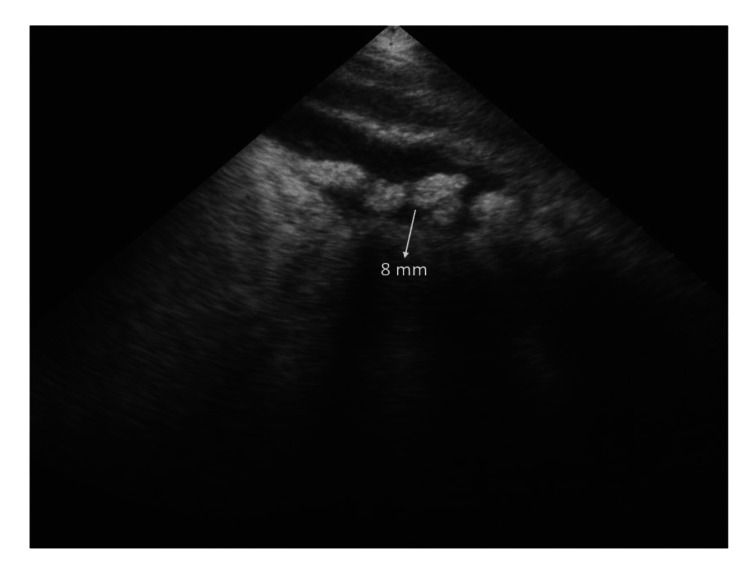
Ultrasound image showing multiple gallstones in the gallbladder
